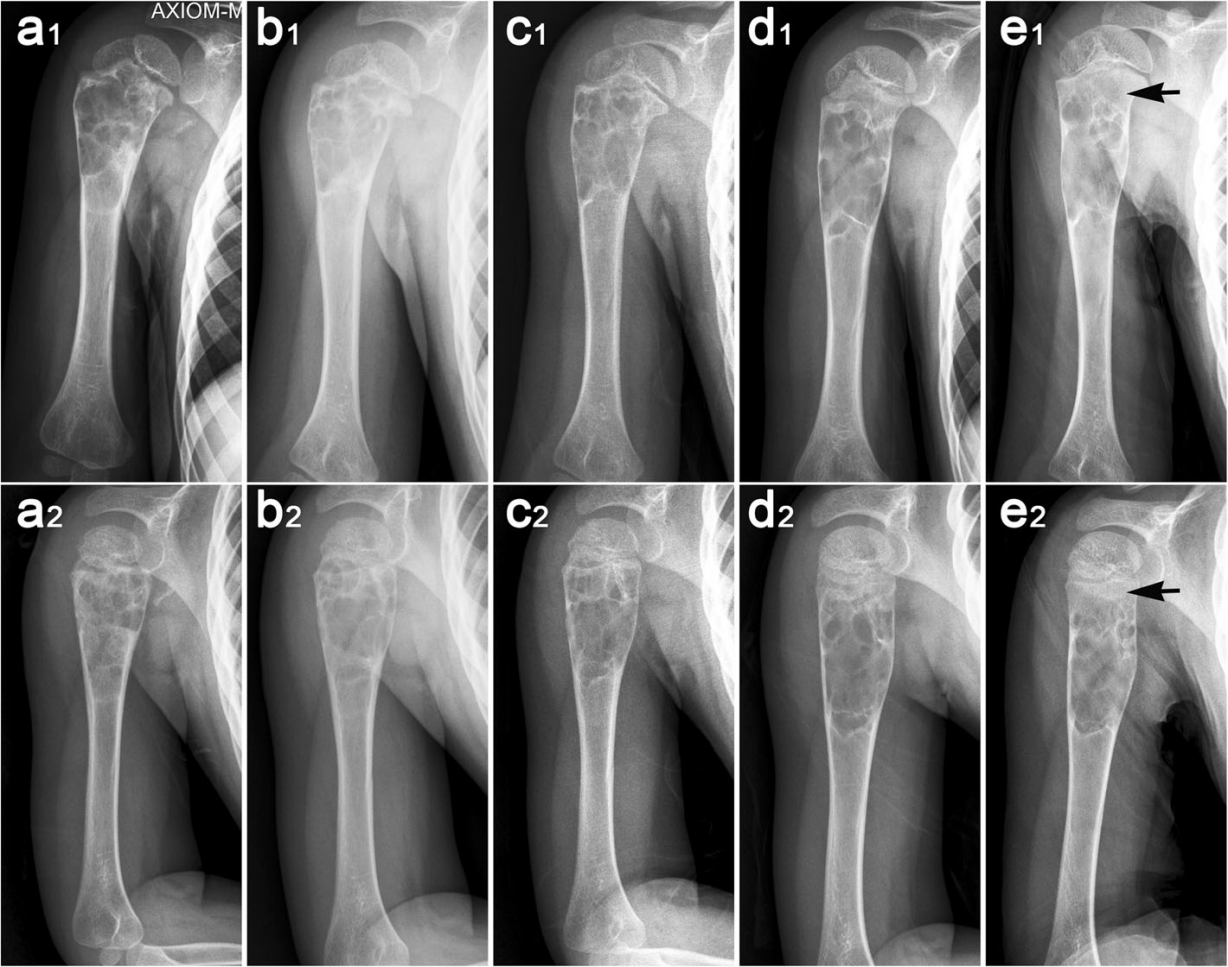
X-ray films of the persistent cysts in a 10-year-old boy after two treatments (1, frontal X-ray; 2, lateral X-ray). a An active unicameral bone cyst was found at the proximal humerus due to pathological fracture and treated conservatively for 6 weeks. b Two months after the first treatment, no significant change was noted in the cyst. c After 2 months of observation, the cyst remained unchanged and was treated for the second time. d Three months after the second treatment, the cyst was partially healed and began to move away from the epiphyseal growth plate. e Nine months after the second treatment, the radiolucent area filled > 50% of the diameter of the bone and the cyst persisted
